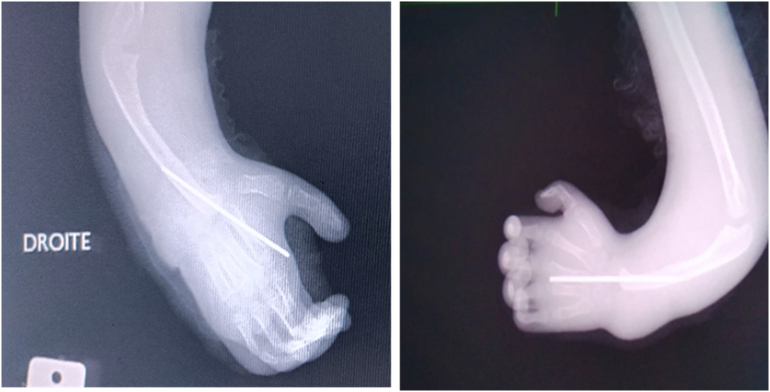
Follow-up radiographs at 3 months postoperatively, prior to pin removal, showing no secondary displacement or pin migration in either hand.
